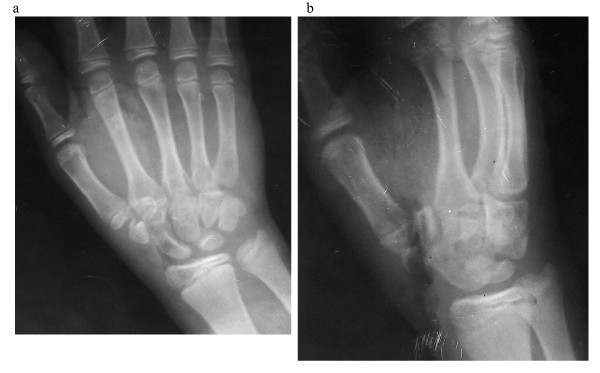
Radiographs of the above patient before surgery.
